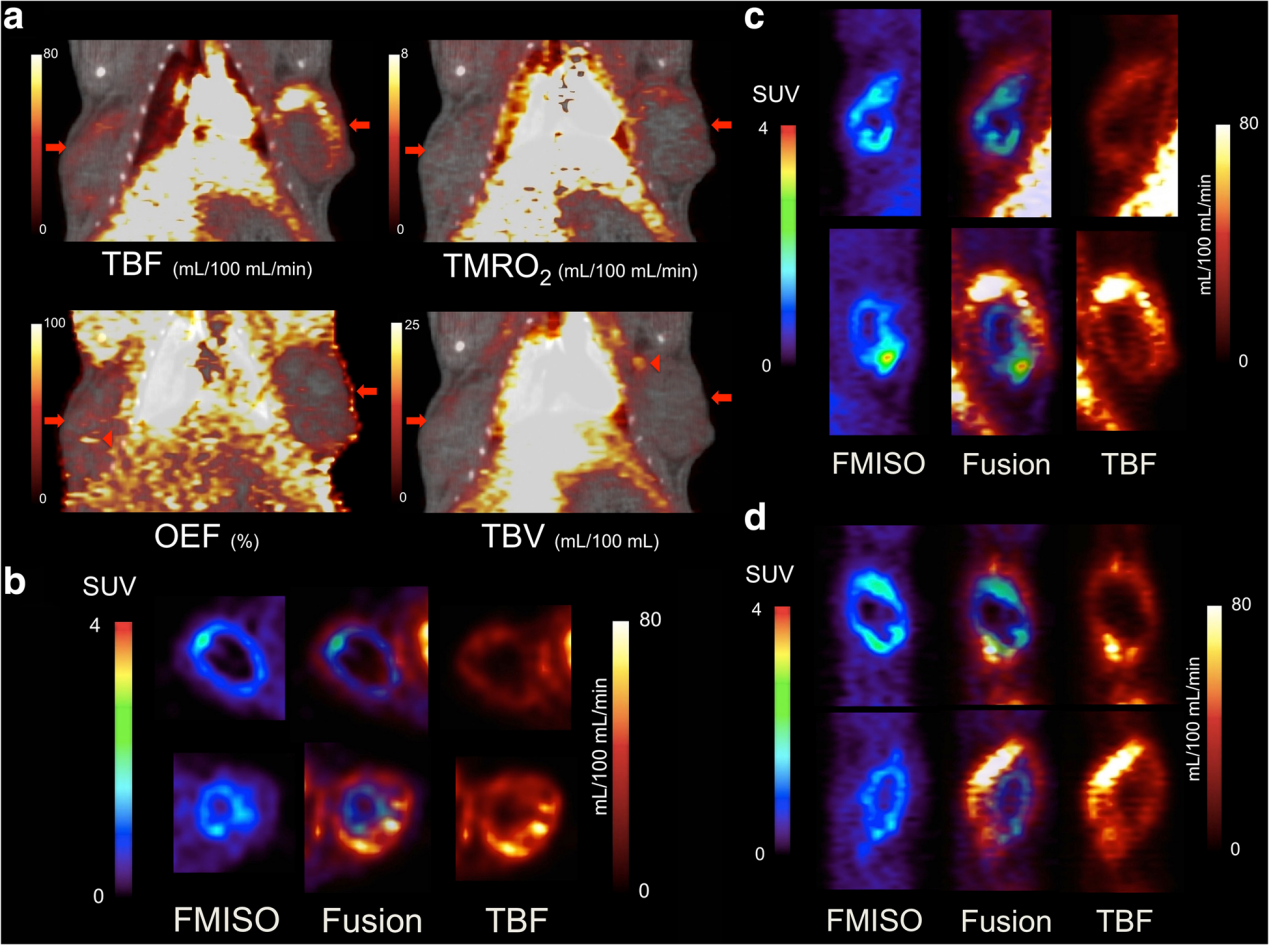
a Functional images of ^15^O-labelled gases PET using 6-min data during steady-state acquisition (coronal slices of PET/CT fusion). TBF and TMRO_2_ images showed marginal dominant distribution (*arrow*). OEF images showed partial elevation in the area close to the center (*arrow head*) and TBV images showed a high spot in the marginal zone of the tumor (*arrow head*). b Axial, c coronal, and d sagittal images of ^18^F-FMISO PET (*rainbow color scale*), TBF PET (*fire color scale*), and co-registered fusion images. The upper figures are tumors in the right side and the lower figures are tumors in the left side
